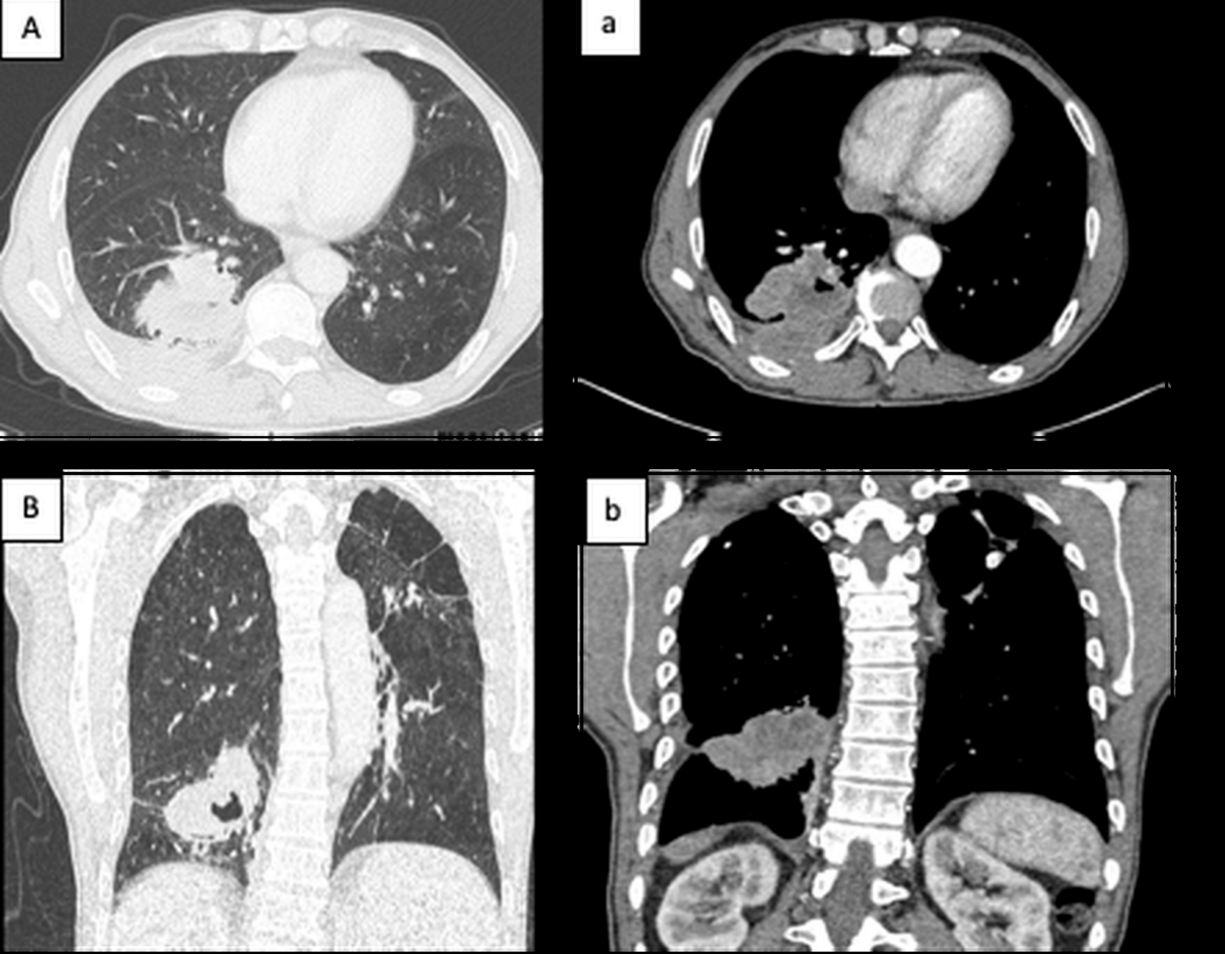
CT scan in coronal and axial parenchymal windows of the lesion process. Axial CT scan in parenchymal window (A) and mediastinal window (a) showing a right Fowler's lobe mass with cavitation and heterogeneous enhancement after contrast administration, coronal CT scan in parenchymal window (B) and mediastinal window (b) showing a right Fowler's lobe mass with cavitation and heterogeneous enhancement after contrast administration.
